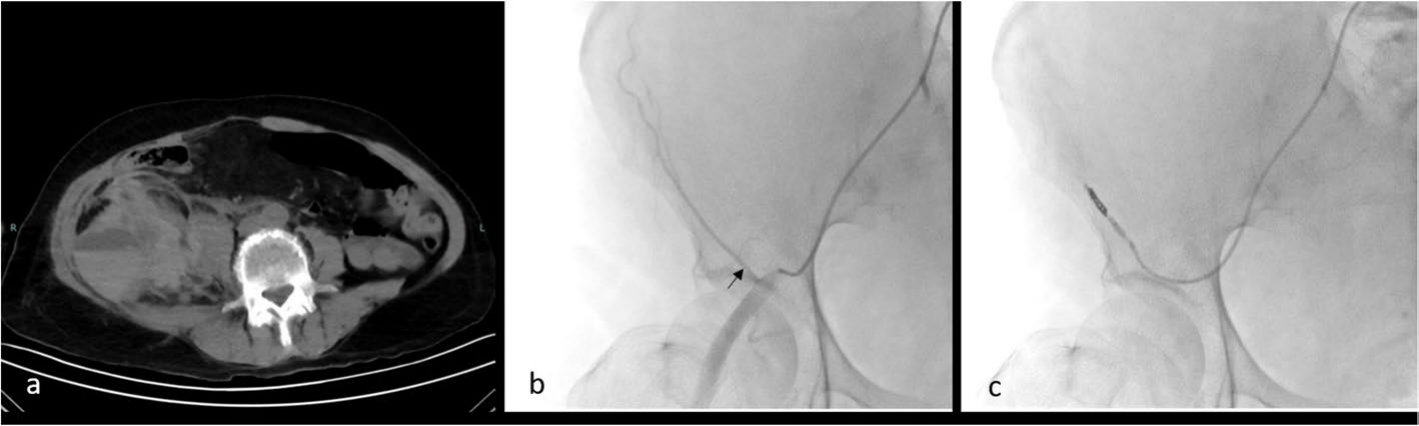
Radiological images of a 73-year-old female patient with a right iliopsoas hematoma. Non-contrast computed tomography image (a). The right external iliac arteriogram revealed the ostium of the deep circumflex iliac artery (black arrow), which was accessed via a coaxial microcatheter (b). Despite no evidence of active bleeding, empirical embolization was performed using coils due to the patient's clinical condition (c)
